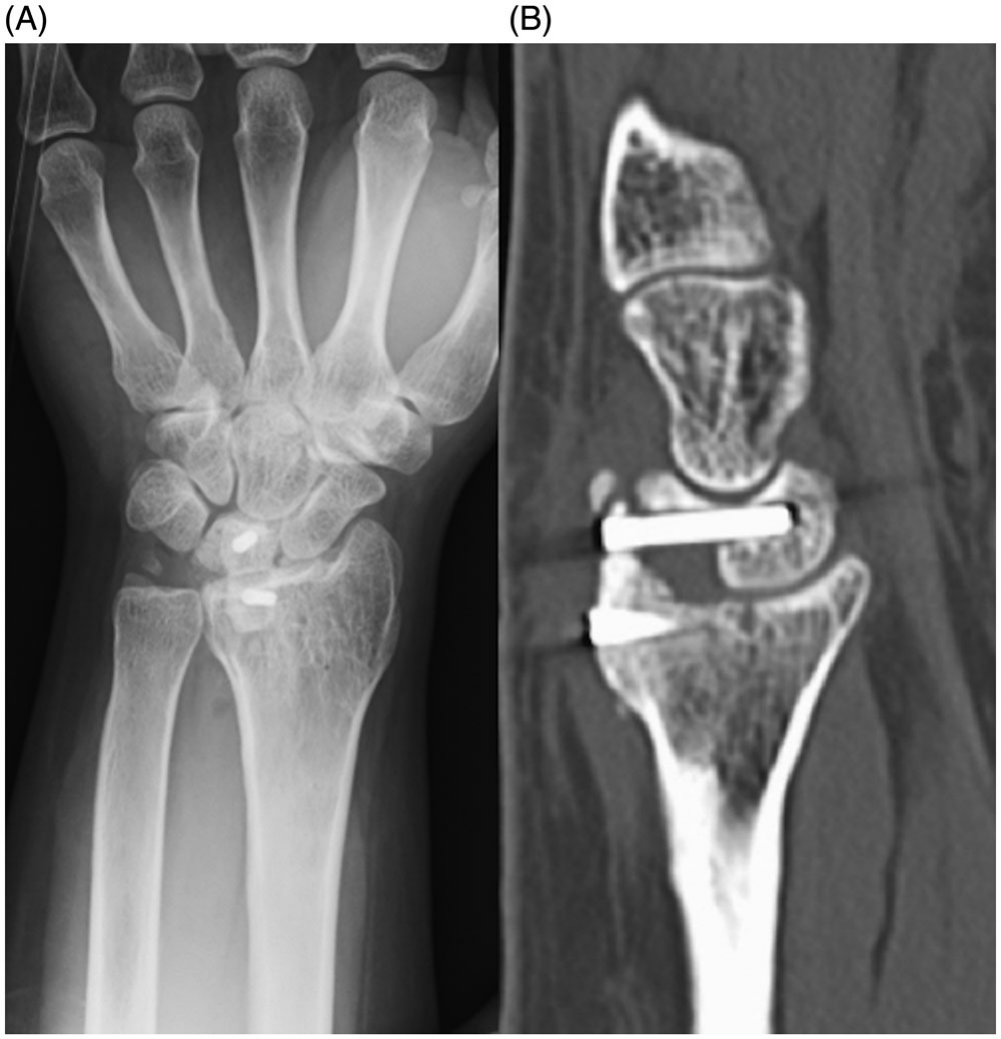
(A) Immediately postoperative radiograph of the radiolunate arthrodesis with the iliac crest. (B) Radiograph at 6 months after the first radiolunate arthrodesis showing nonunion.
